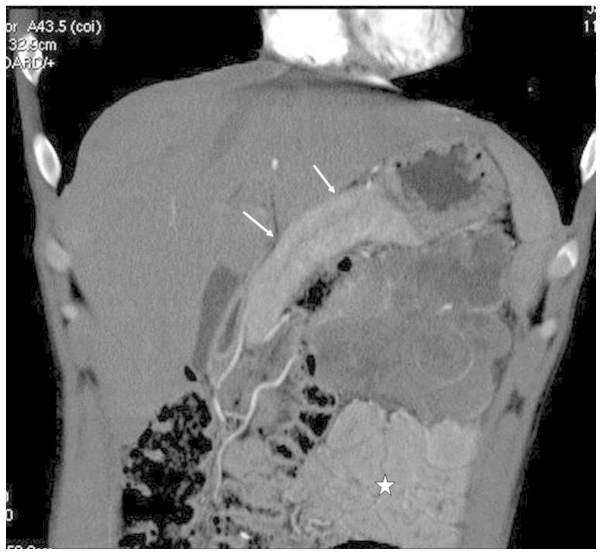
Coronal reconstruction image from contrast-enhanced computed tomography demonstrates the association between the mass and the bowel loops and the pancreas. It constricted and distorted the pancreas (shown by the arrow) and bowel loops (shown by the star).
